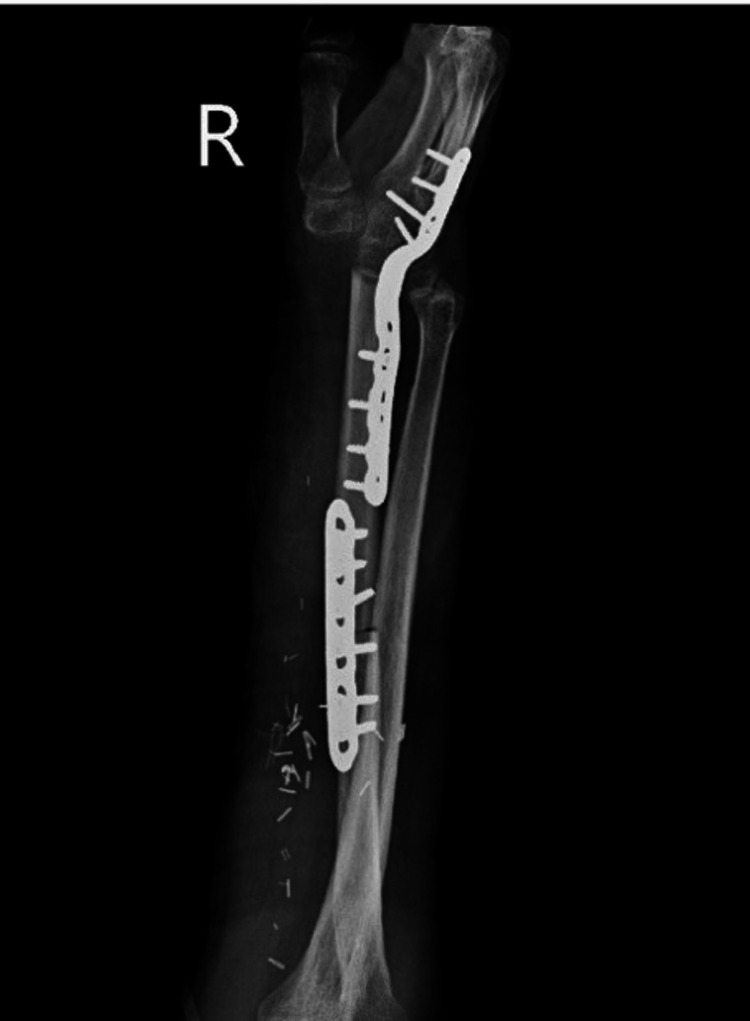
Immediate postoperative radiograph of the forearm demonstrating reconstruction with a vascularized fibular flap, stabilized using a fixation system.
